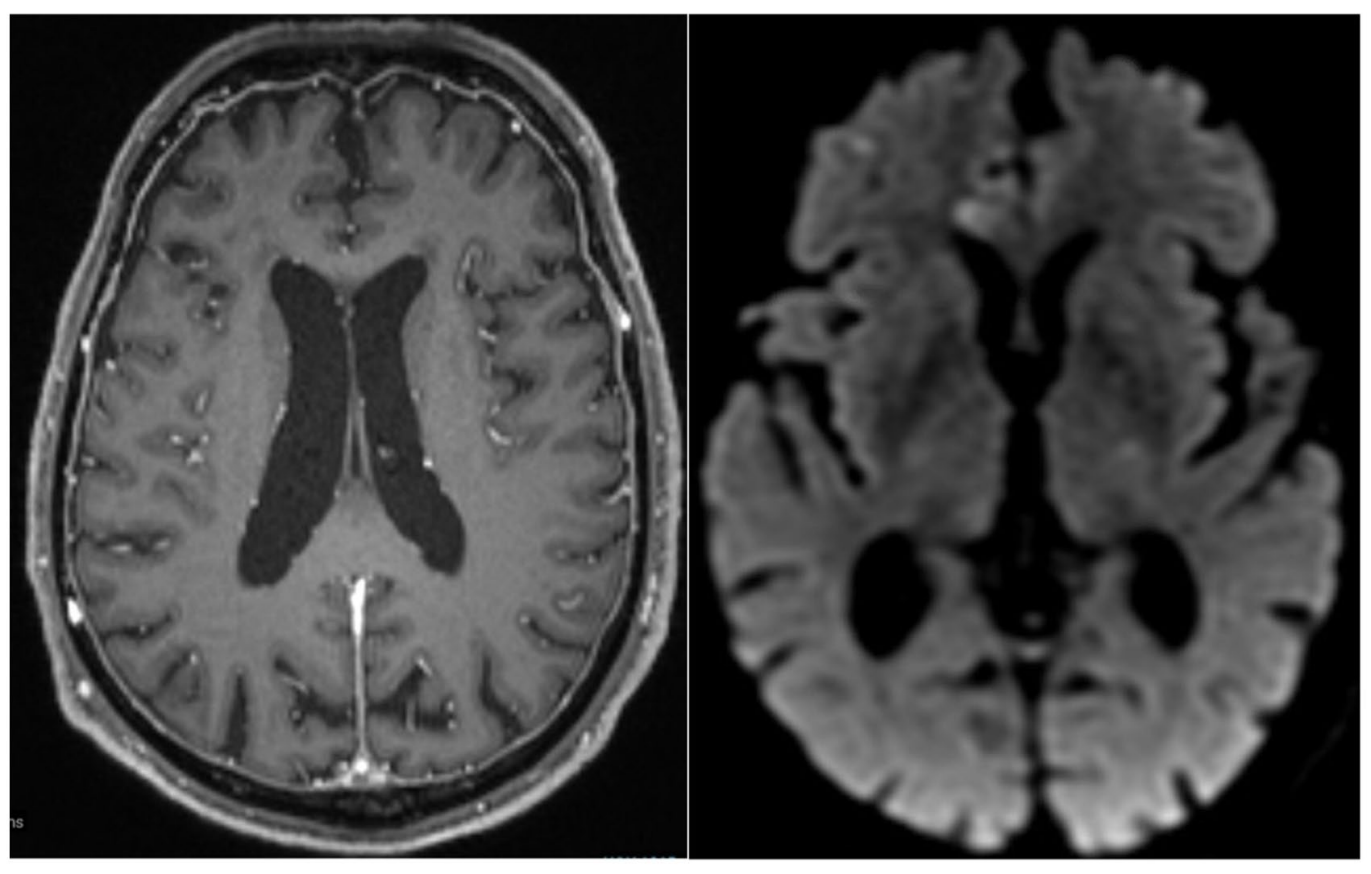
Case 1 MRI: diffuse pachymeningeal enhancement (T1 + GAD, Left); Lacunar infarcts (DWI, Right).
